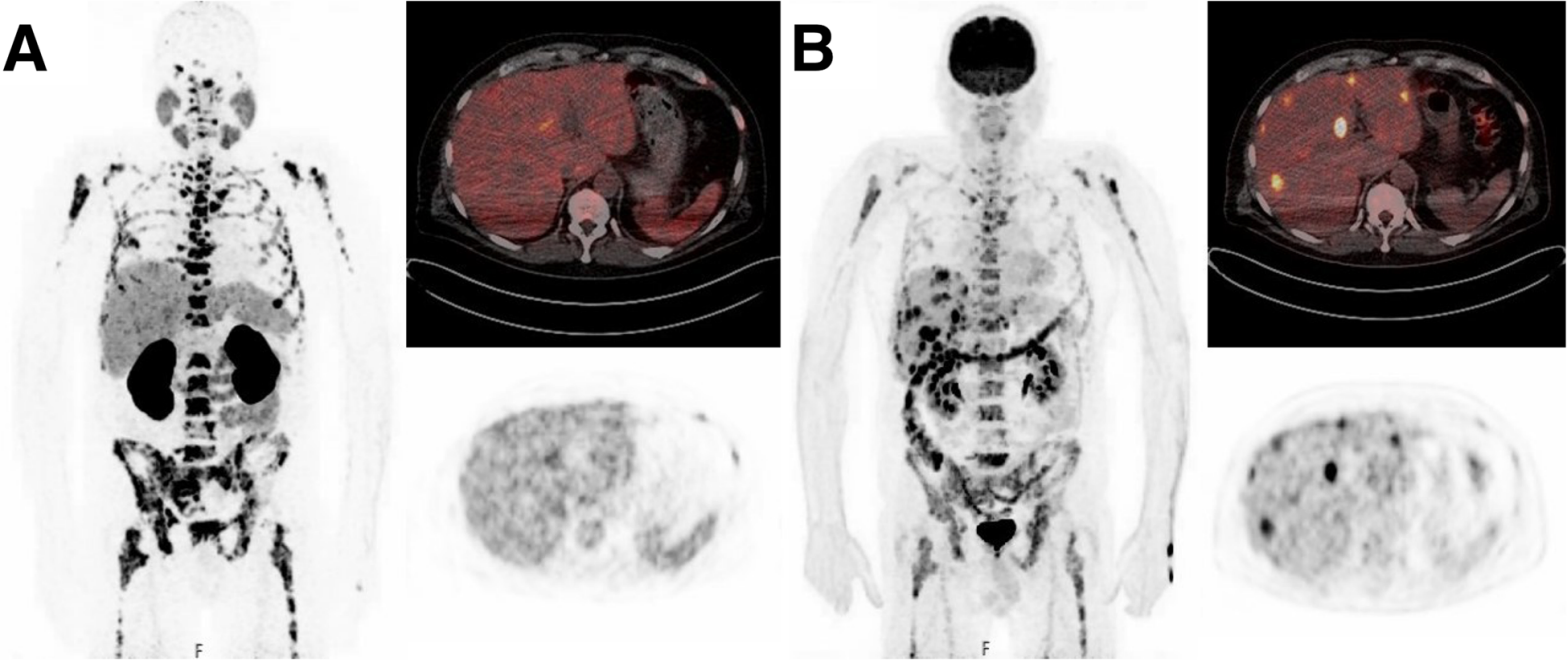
Patient with hepatic mismatch findings. Maximal intensity projection (MIP), PET/CT, and PET data of [^68^Ga]Ga-PSMA-11 PET/CT (a) and of [^18^F]FDG PET/CT (b)
